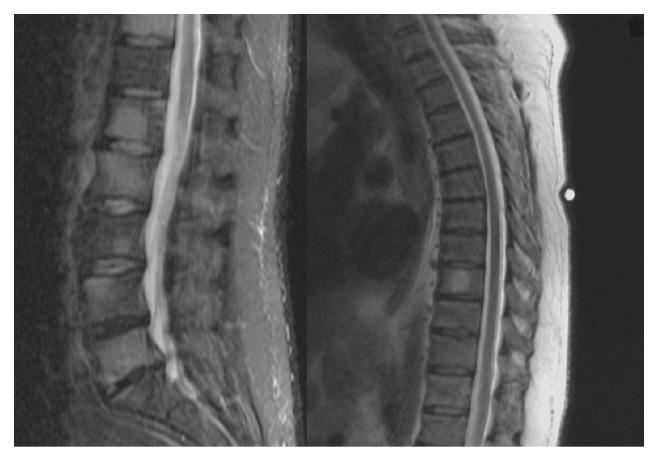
MRI T2 images showing thoracic and lumbar vertebral metastatic disease.
